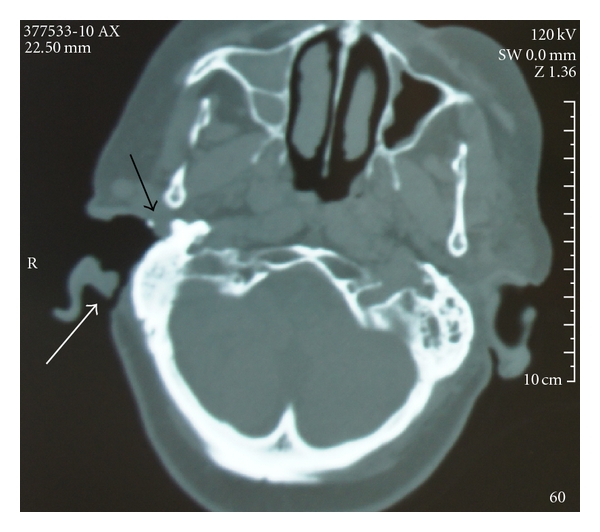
Axial high-resolution CT scan showing the retroauricular fistula (white arrow), sclerotic mastoid and granulation tissue in the middle ear area (black arrow).
